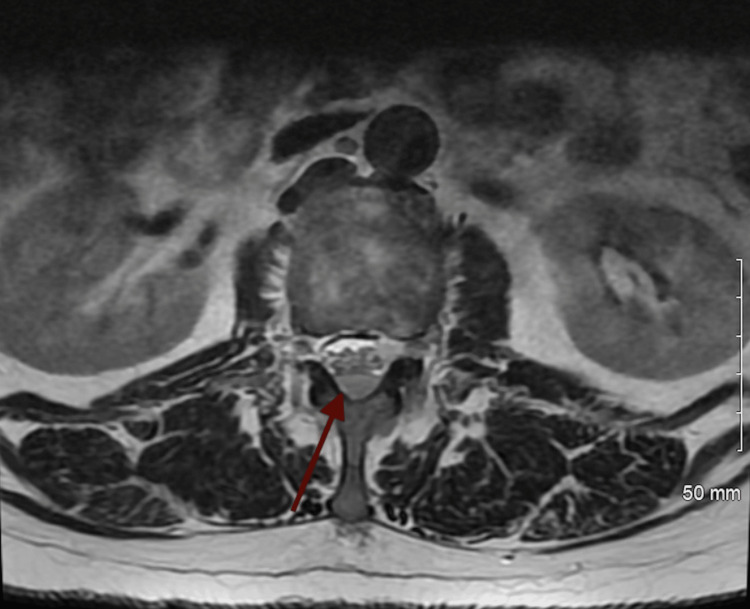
MRI imaging showing mild enhancement of the cauda equina nerve roots, suspicious for carcinomatosis The red arrow depicts the area of enhancement, shown as white discoloration of the cauda equina, demonstrating malignant carcinomatosis. MRI: Magnetic resonance imaging
